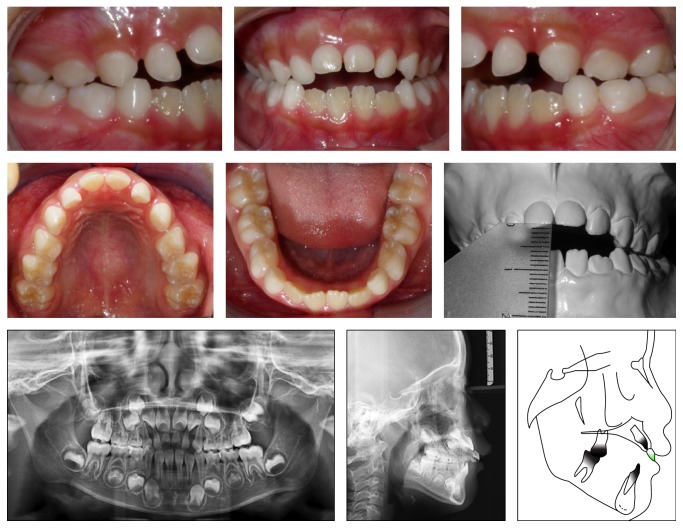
Pretreatment intraoral photographs, radiographs, and cephalometric tracing.
